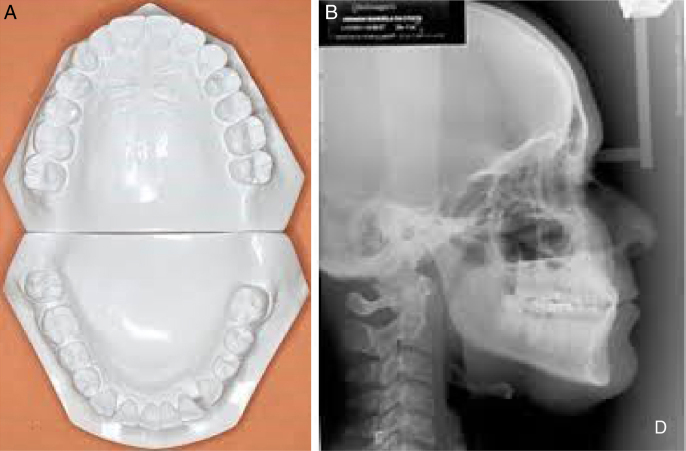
A, Study models used for assessment. These models were scanned so that the digital measurements could be performed. B, Radiographs used to assess the position of the incisors.
